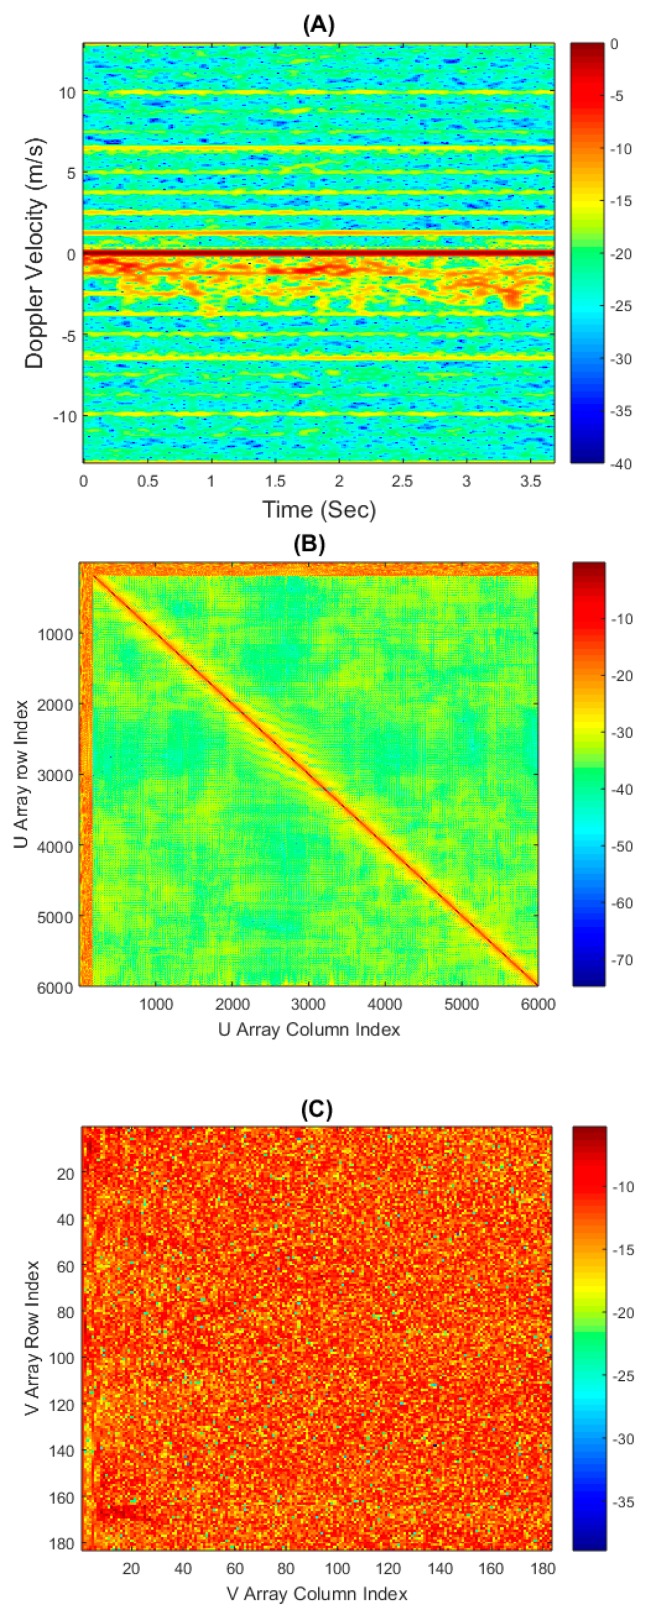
(A) micro-Doppler section from free handed motion; (B) Singular Value Decomposition (SVD) *u* Array plotted as 10×log10(u) from micro-Doppler section; (C) SVD *v* Array plotted as 10×log10(v) from micro-Doppler section.
